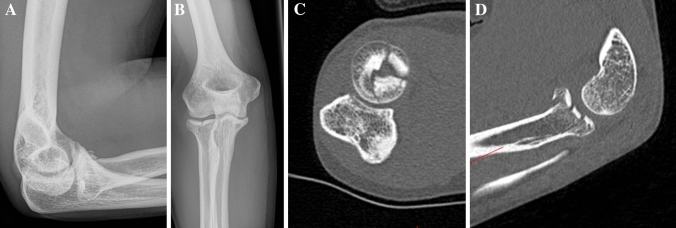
a, b Preoperative radiographs (lateral and anteroposterior) of a Mason type III fracture (Case1). c CT-scan in axial view and d in sagittal view showing the mutifragmental radial head
